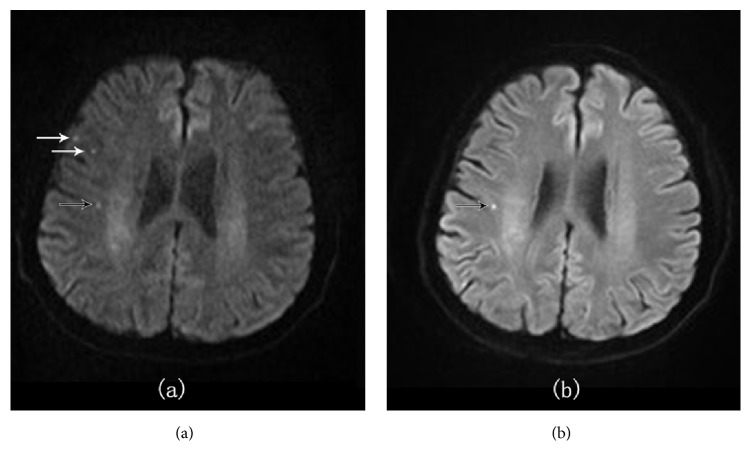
A 49-year-old male patient with acute cerebral infarction. (a) There are 3 suspicious subtle lesions at the right frontal cortex on routine ss-DWI. (b) Only 1 subtle lesion (black arrow) appeared in rs-DWI. Two lesions (white arrows) on ss-DWI did not appear in rs-DWI. rs-DWI showed increased specificity to ss-DWI.
